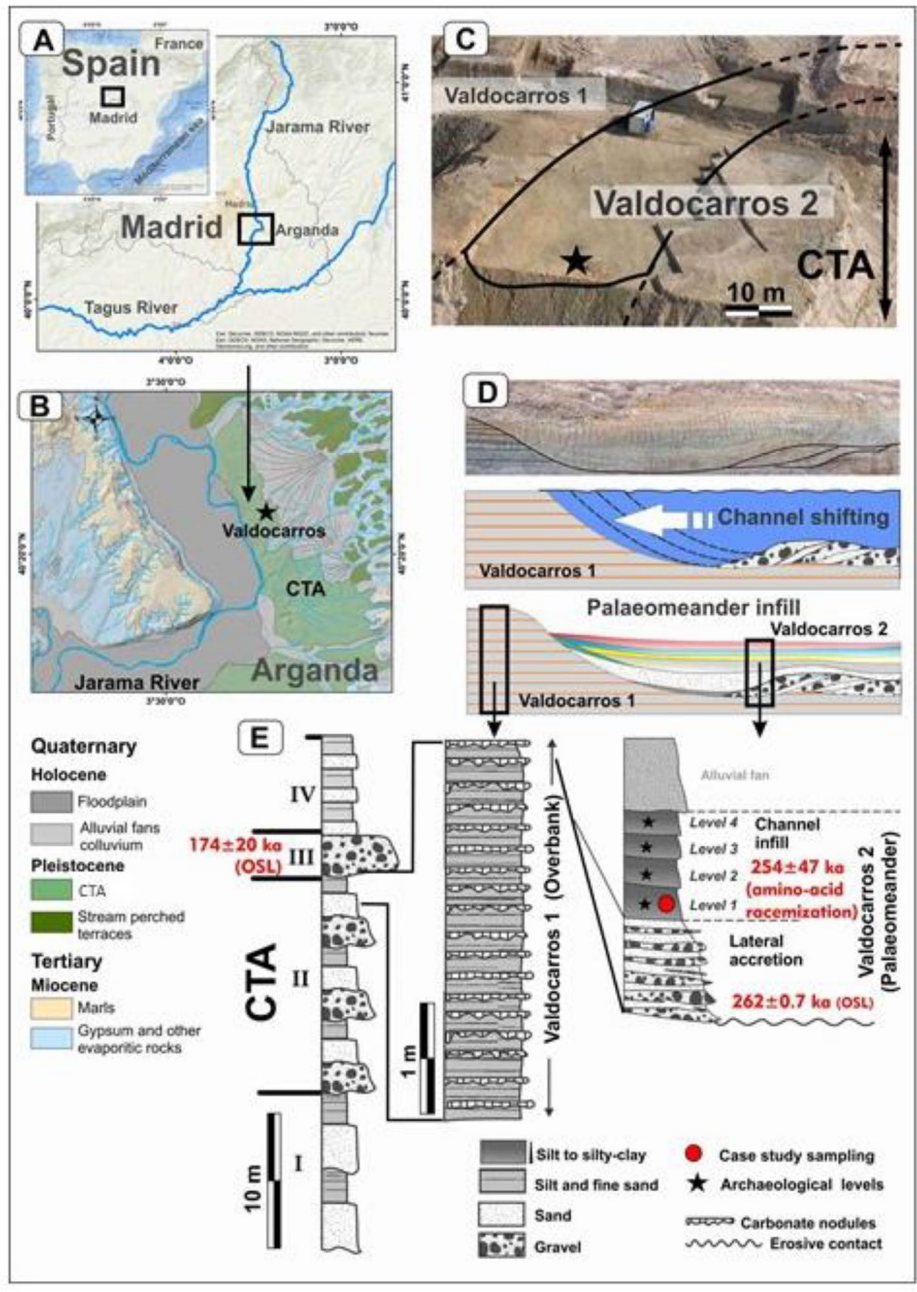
Study area and sample location. (A) Geographical location of Valdocarros in Spain, Madrid. (B) Lithology and geomorphology. (C) Aerial view of the gravel pit in the Complex Terrace of Arganda (CTA) and Valdocarros 2 site. (D) Evolution of Valdocarros 2 palaeomeander in previous overbank units. (E) Stratigraphy, chronology, and sampling. This figure was made using ArcGis 10.6.1. https://www.esri.com/fr-fr/arcgis/products/arcgis-desktop/resources by David Uribelarrea.
